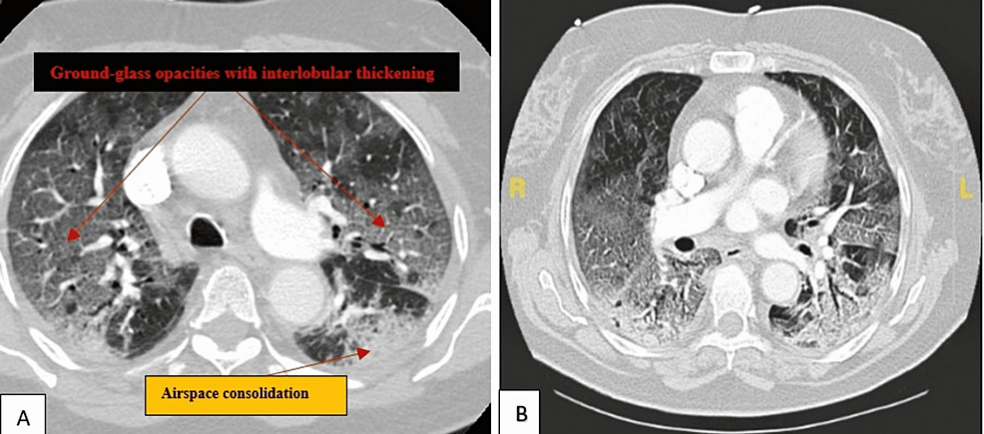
Image A and B shows axial view of the chest CT lung window demonstrating ground-glass opacities with interlobular septal thickening and bibasilar airspace opacities.
